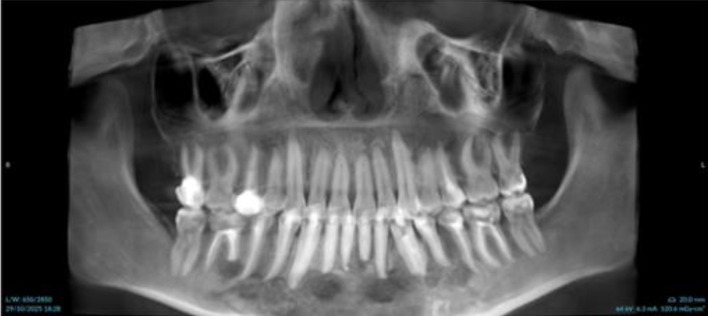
The 3-month cone beam computed tomography follow-up.
